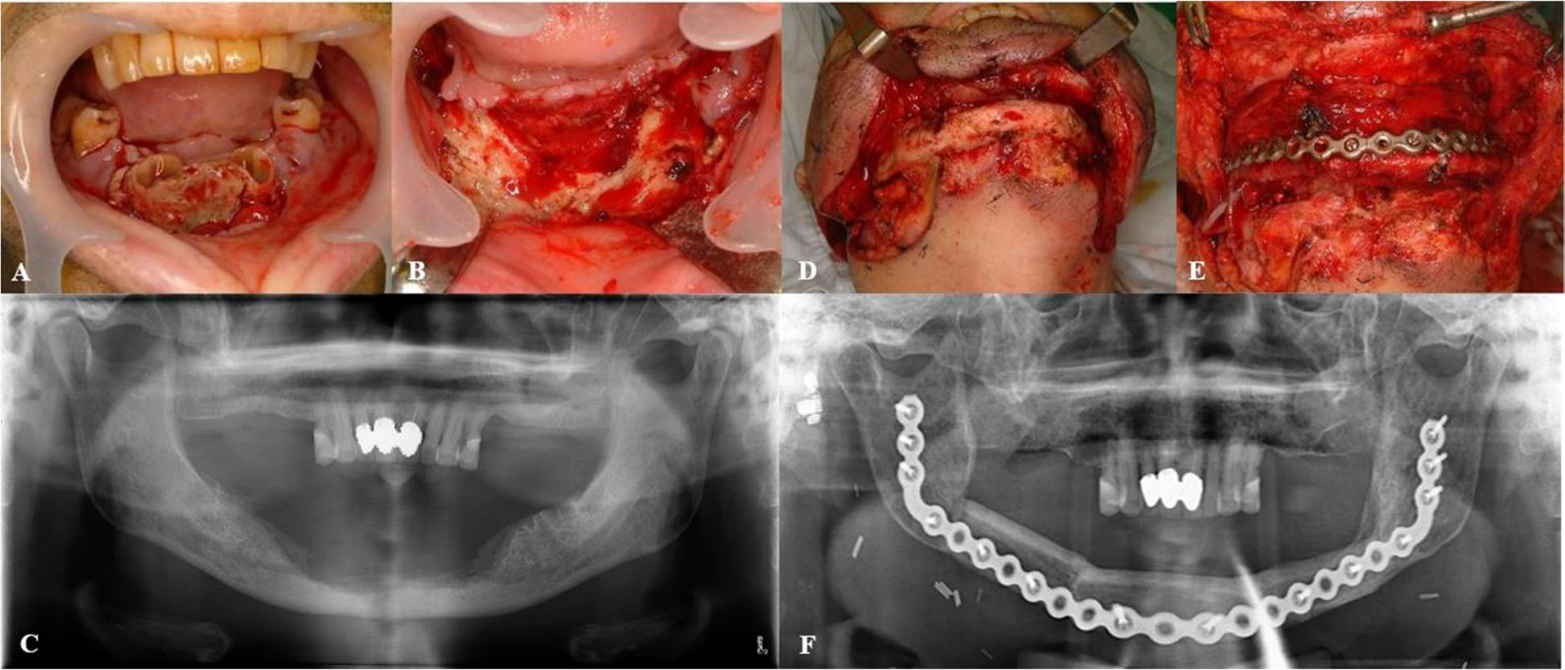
Surgical treatment of medication-related osteonecrosis of the jaw in multiple myeloma patient. A Preoperative state showing exposed bones anterior mandible. B Saucerization and extraction performed. C Postoperative panoramic radiograph after 1day of saucerization. D Progressed disease in the mandible. E Reconstruction of the mandible with fibular free flap and reconstruction plate. F Postoperative panoramic radiograph after 2 years showing stable results
